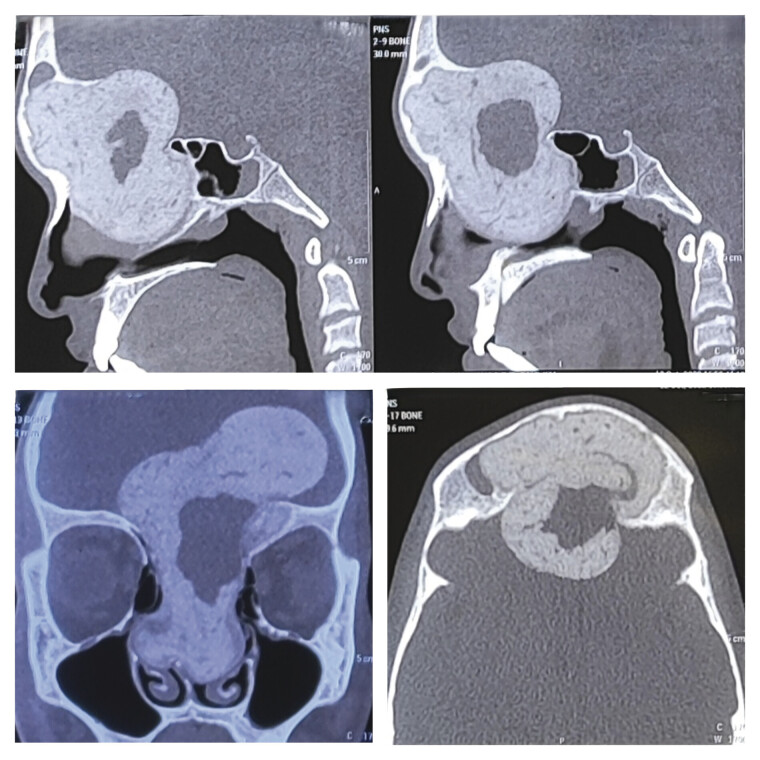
Computed tomography (CT PNS) clockwise—sagittal, sagittal, axial, and coronal images showing a well-defined, multilobulated, heterogeneously hyperdense lesion involving the ethmoid sinuses, frontal sinus almost entirely, also extending into the cranial cavity into the anterior cranial fossa. The lesion also has a hypodense cystic component in its center. PNS, para nasal sinuses.
